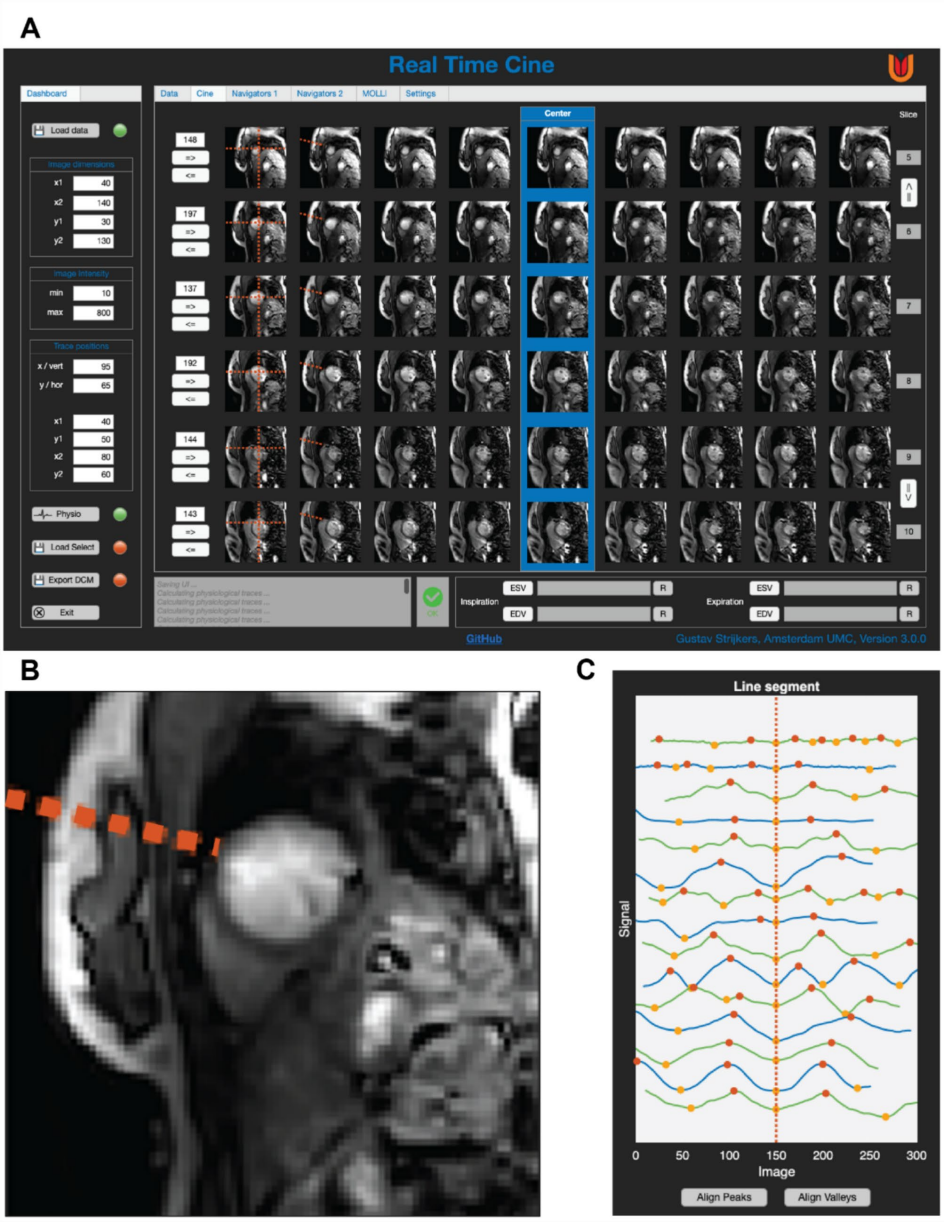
Screenshot of MATLAB-based user interface for retrospective frame sorting (A). A ‘navigator’ line (red line) is defined manually over the chest-lung interface (B). The mean signal intensity over this line is then plotted against time (C), with detection of peaks (orange dots) and valleys (ocher dots) that reflect end-expiration and end-inspiration phases, respectively. Each trace (300 frames; 10 s) represents a slice in the stack of real-time cine MRI series acquired from apex (top) to base (bottom) during free breathing. Respiratory rate in this example of moderate-intensity bicycling exercise was approximately 15 breaths/minute (i.e., 2.5 respiratory cycles/trace), although some variations in breathing frequency occurred. Respiratory phases could not be visualized clearly in more apical slices due to less motion of the chest or abdomen in these images, resulting in low or absent signal intensity peaks or valleys. Buttons allow the user to respiratory phase-align all slices based on detected peaks or valleys, retrospectively synchronizing respiration between consecutively acquired slices in the stack of real-time cine MRI series. Then, the user can define the end-systolic and end-diastolic phase per slice by positioning the corresponding frame in the ‘center’ (blue box). The resulting images are aligned to both the cardiac as well as the respiratory phases, and can be exported in their original DICOM format for volumetric analyses. The software is available on GitHub (https://github.com/Moby1971/RealTimeCine-v3.0)
